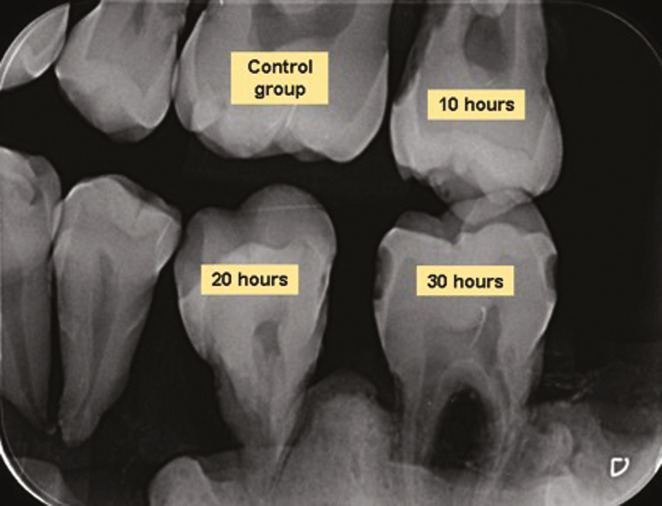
Interproximal X-ray of sampled teeth.
